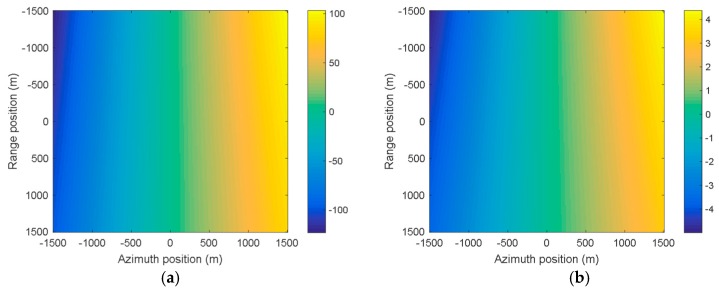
The spatially-variant phase errors caused by the spatially-variant Doppler parameters. (a) The phase error caused by the Doppler rate; (b) the phase error caused by the derivative of the Doppler rate.
